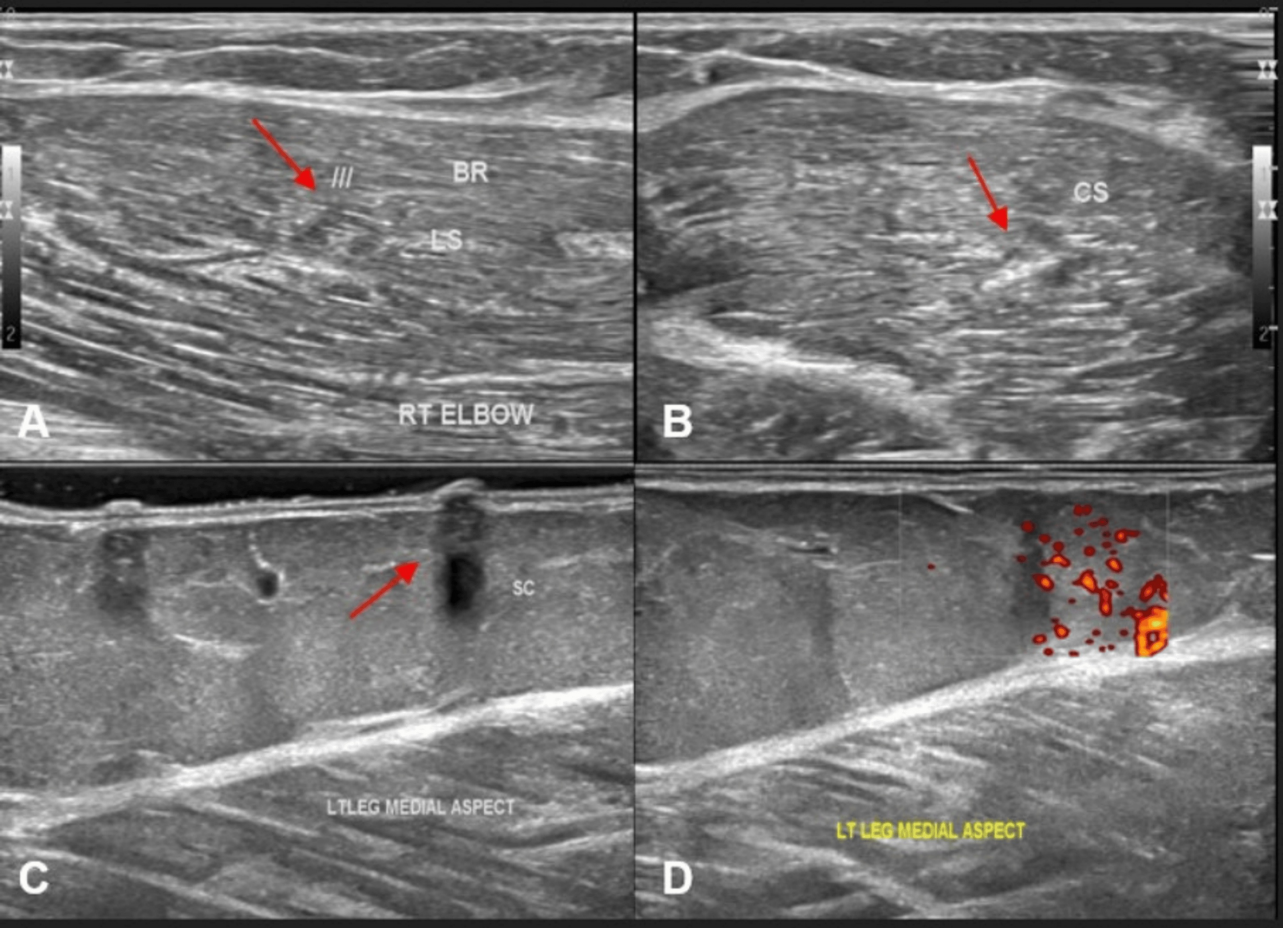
Ultrasound of right elbow (A, B) and left leg (C, D) showing soft tissue cysticercosis Ultrasound images of the right elbow in longitudinal section (A) and cross-section (B) showing a well-defined oval hypoechoic area (indicated by red arrows) measuring ~5 x 5 mm seen in the brachioradialis muscle - likely uncalcified cysticercosis. Ultrasound images of the left leg in longitudinal section (C) showing an irregular hypoechoic area (indicated by red arrow) measuring ~7 x 4 mm in the subcutaneous plane, with increased vascularity on power Doppler imaging (D) - likely inflammatory changes in the subcutaneous plane.
